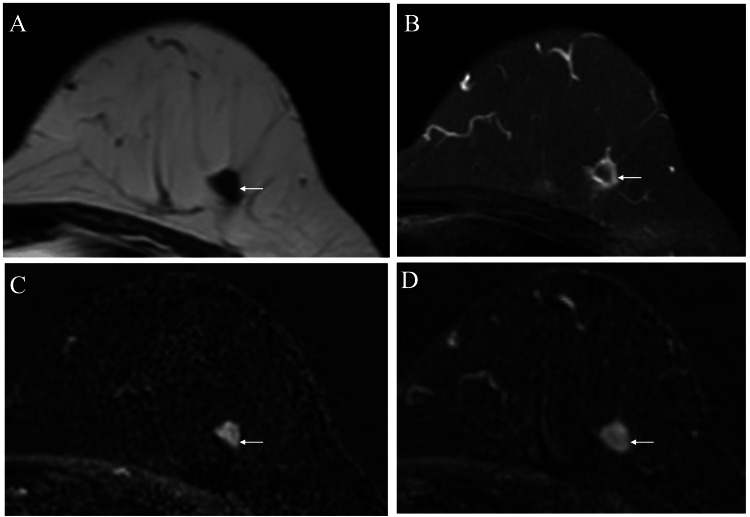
Magnetic resonance imaging (MRI) findings of the tumor (A) MRI of the tumor (arrow) showing a hypointense pattern on T1-weighted images. (B) MRI showing a hypointense mass encircled by a hyperintense rim (arrow) on fat-suppressed T2-weighted images. (C) Time-signal intensity image showing a weak enhancement (arrow) on early arterial phase images. (D) Time-signal intensity image showing a retained weak enhancement (arrow) on delayed phase images.
